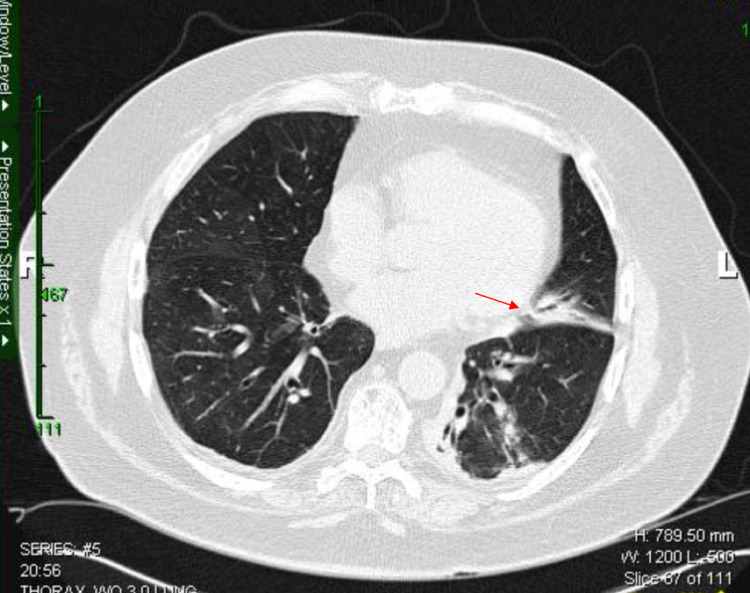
CT scan of the thorax showing the focal area of consolidation (red arrow)
